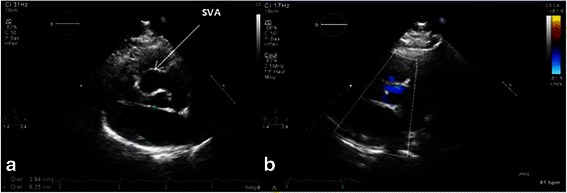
Echocardiography. a Showing the Sinus of Valsalva aneurysm (SVA) extending into the right ventricle. b Color Doppler showing the aortic regurgitation
